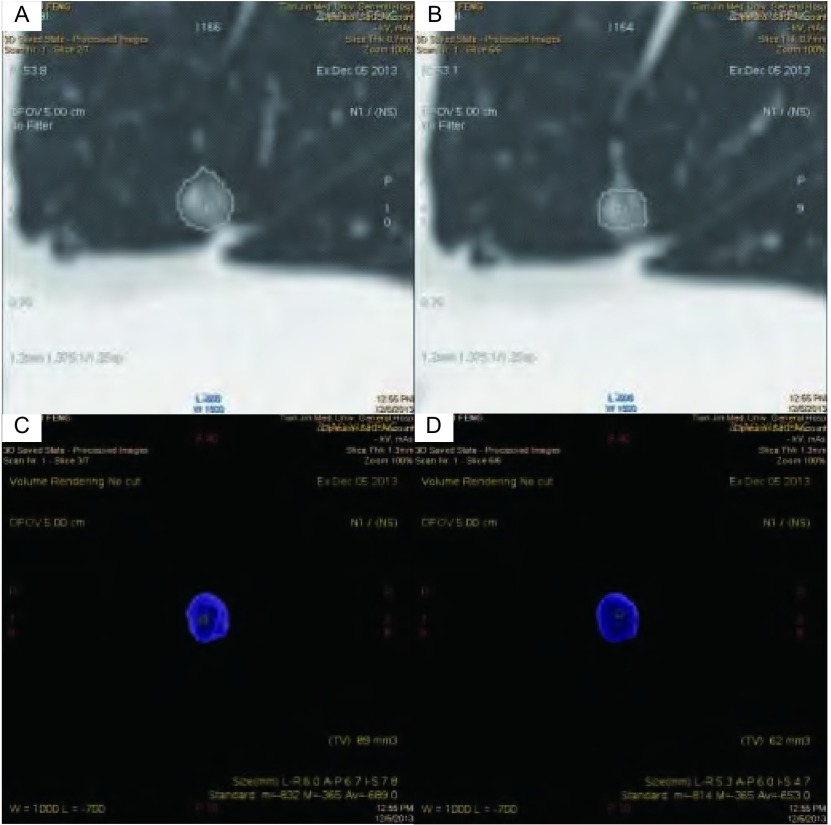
女，53岁，右中叶磨玻璃密度结节，直径约为4.6 mm。图A、C为观察者1第一次测量，大小为89 mm^3^，密度为311 mg/cm^3^，质量约为27.679 mg。图B、D为观察者1第二次测量，大小为62 mm^3^，密度为347 mg/cm^3^，质量约为21.574 mg。质量变异程度与体积变异程度分别为25.0%、35.8% Volume and attenuation measurement of GGN in a 53-year-old woman by using semiautomatic software program. Thin-section chest CT image in lung window setting shows 4.6-mm GGN in right middle lobe. A, C: the 1st measurement of observer 1, the size of the GGN is 89 mm^3^ and the atteunuation is -689 HU, so the mass is about 27.679 mg; B, D: the 2nd measurement of observer 1, the size of the GGN is 62 mm^3^ and the atteunuation is -653 HU, so the mass is about 21.574 mg. The variation of the mass and the volume is 25.0%, 35.8%
